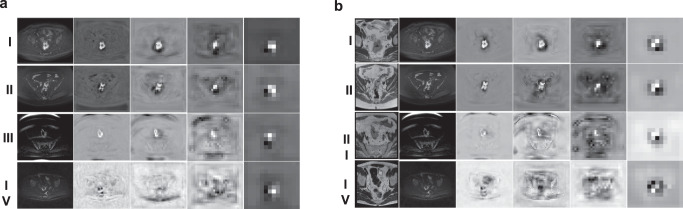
Feature maps visualization. Feature maps derived from nnUNet by deep supervision cases for I−IV. a using DWI alone; (b) using DWI + T2W.
